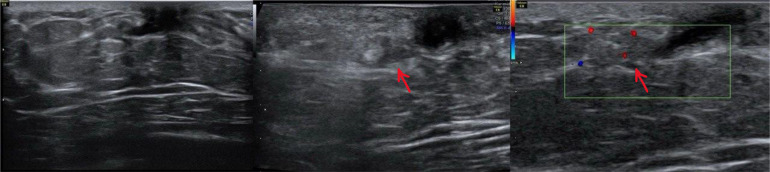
breast ultrasound shows a dilated duct in retroareolar region and an isoechoic lobulated intraductal mass (7 × 3 mm) with central vascularity in the left breast (arrow).
